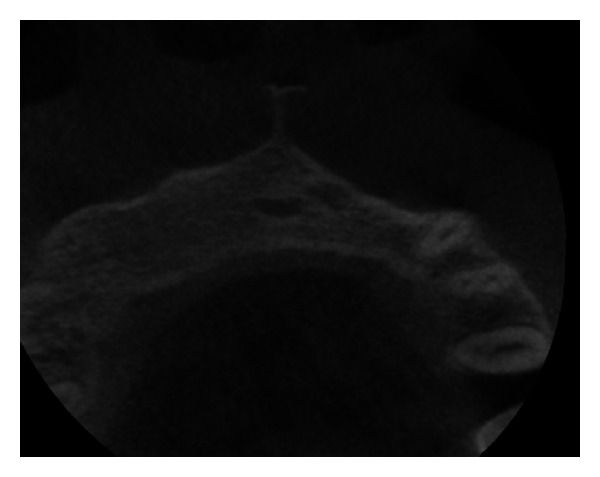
1-year followup CBCT image in axial plane showing establishment of the buccal and lingual cortical plates.
